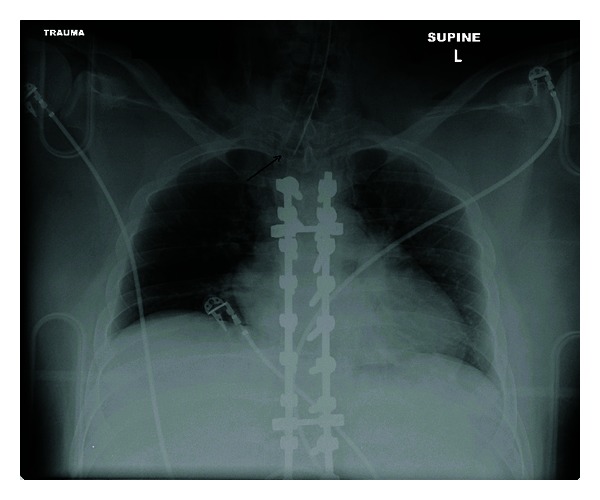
Chest X-ray after reintubation. Arrow: tip of ETT is at the thoracic inlet.
